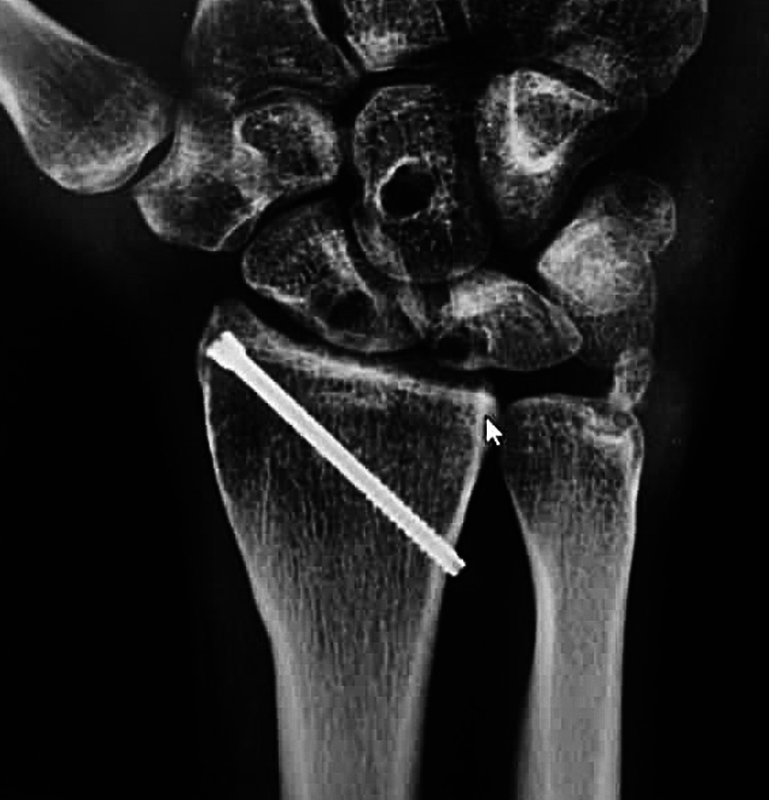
Postoperative aspects of anteroposterior wrist radiography.
